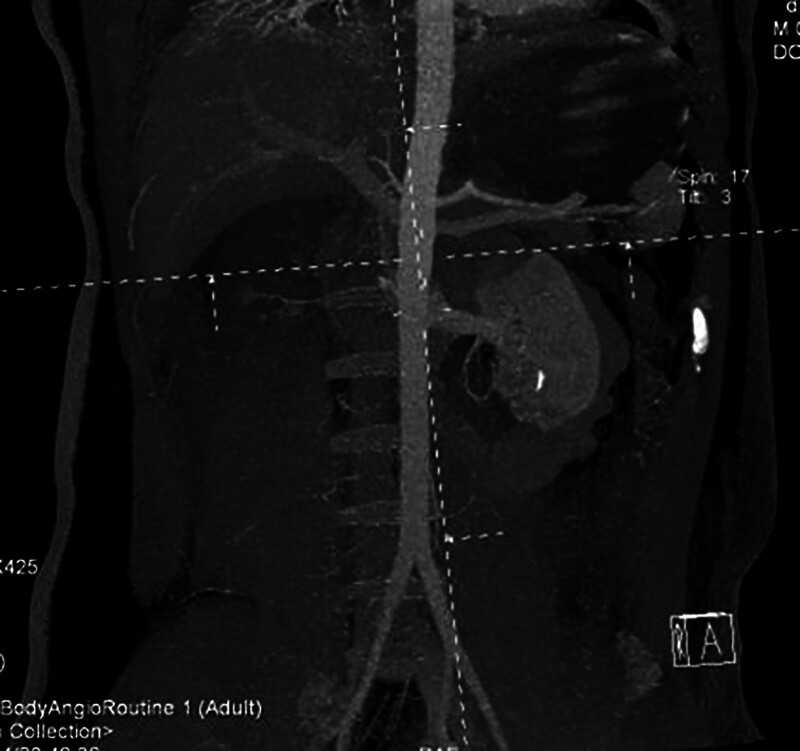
CTA demonstrates poor visualization of the right renal artery.
